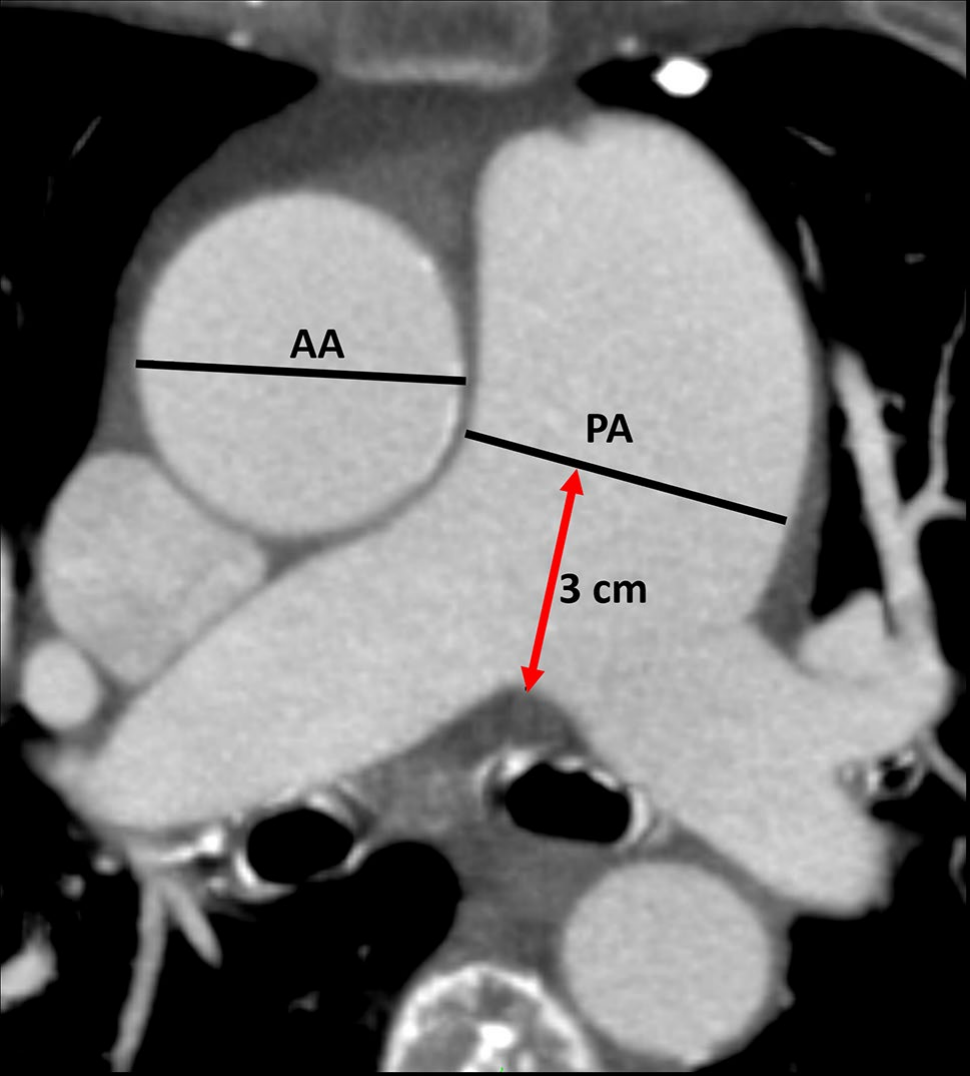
Measurement of diameters of interest on axial CT. AA: ascending aorta; PA: main pulmonary artery; red double headed arrow: distance between bifurcation of the pulmonary trunk and level of measurement within main pulmonary artery
